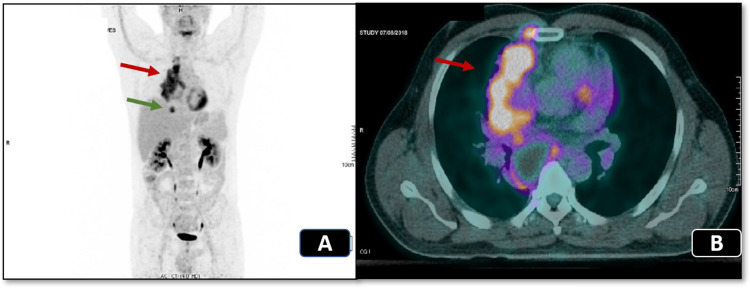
PET CT in August 2018 (A - coronal plane; B - transversal plane) showing the paramediastinal mass (red arrow) and the epiphrenic lymph node (green arrow).
